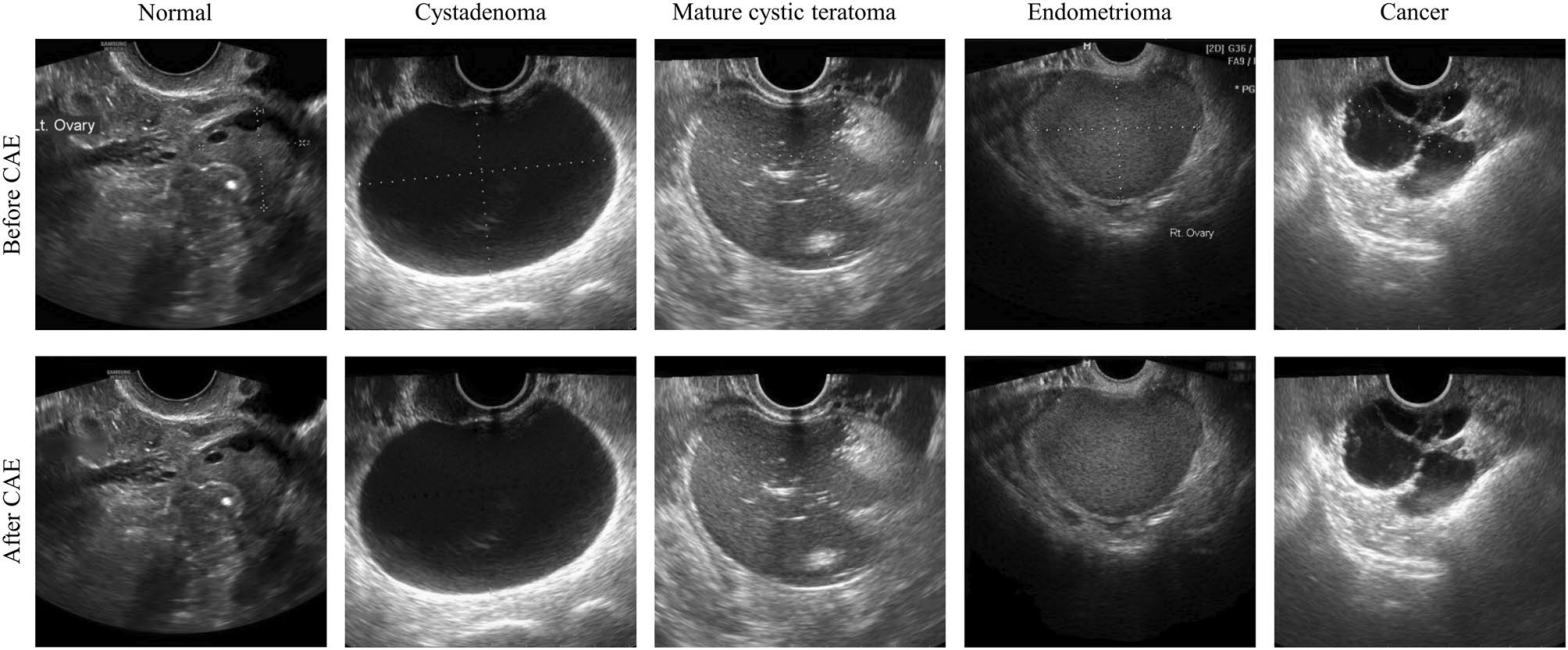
Ultrasound images before and after removing the marks via the convolutional autoencoder. The first row is the images with marks, and the second row is the image without marks. Example images are from left to right normal, cystadenoma, mature cystic teratoma, endometrioma, and malignancy.
